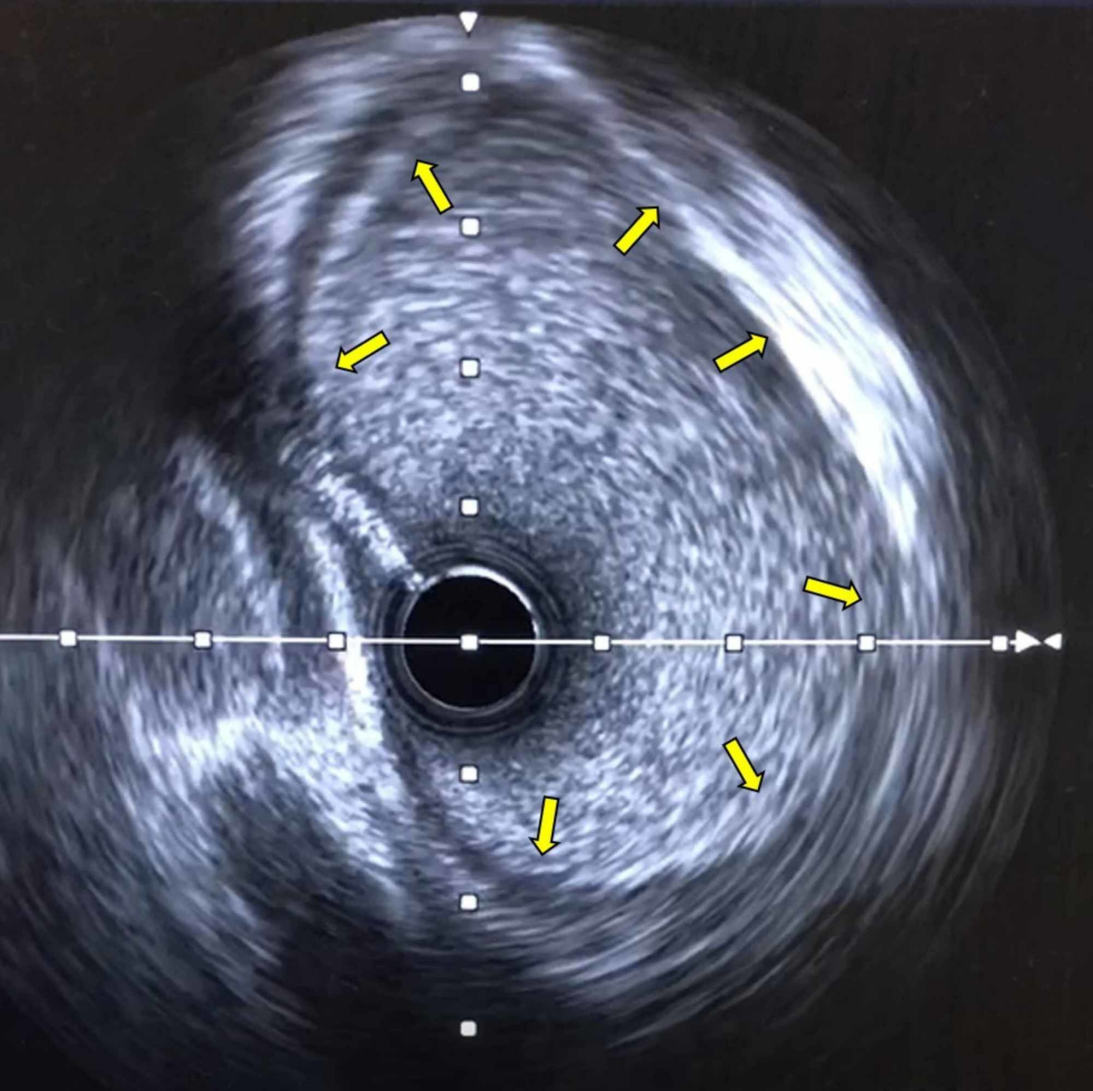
IVUS in our second patient IVUS at the level of LCA showing a lack of intramurality and no evidence of stenosis. IVUS: intravascular ultrasound; LCA: left coronary artery
